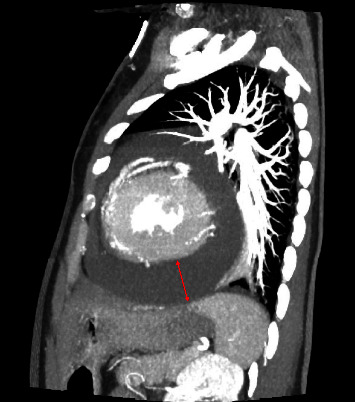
Chest CT with angiography: sagittal view shows large pericardial effusion (red line) without pericardial or pleural thickening or pericardial or pleural masses.
